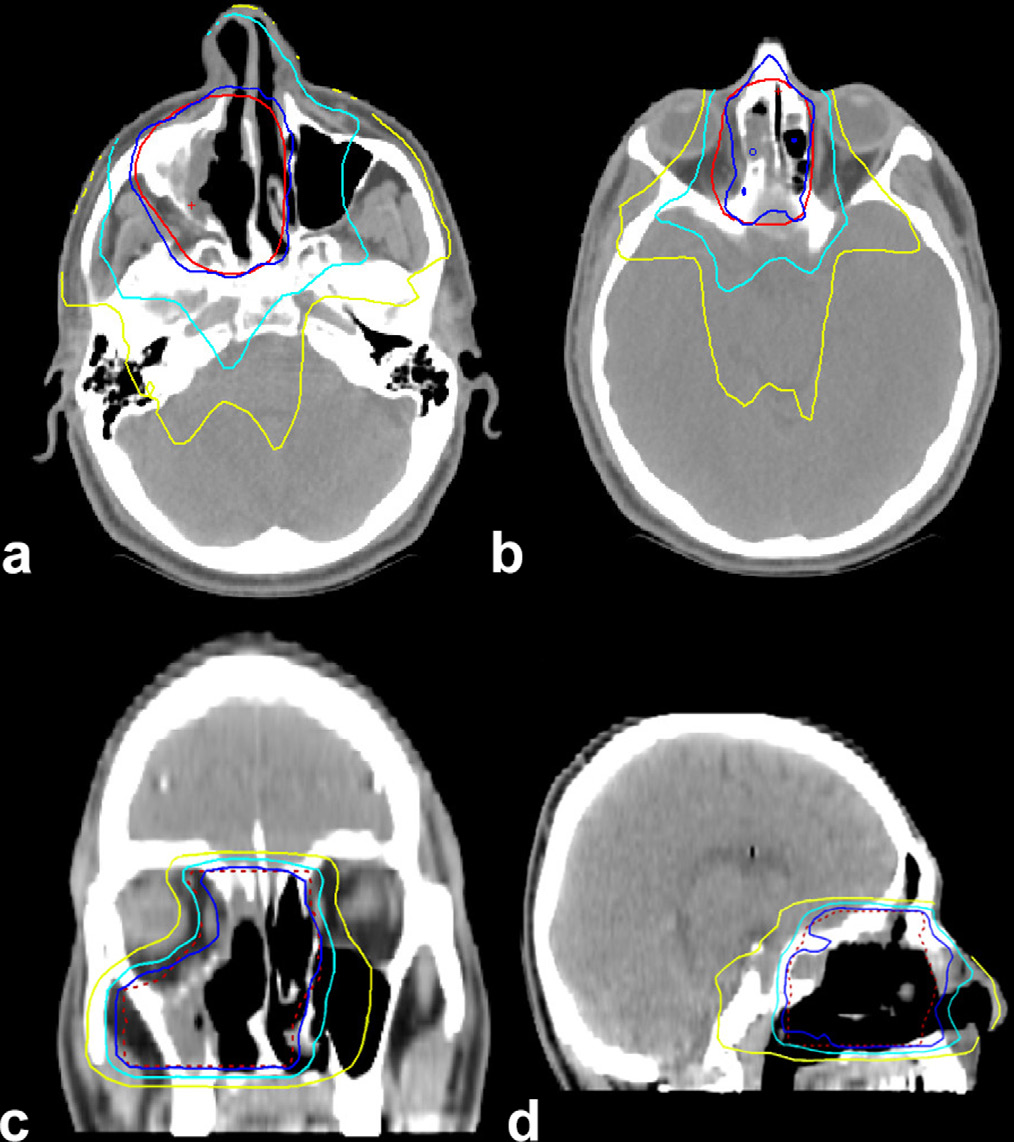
Dose distribution in axial (a, b), coronal (c) and sagittal (d) CT planning slices: the inner line corresponds to the planning target volume, whereas the other lines going outwards represent 95, 80 and 50% isodoses, respectively
